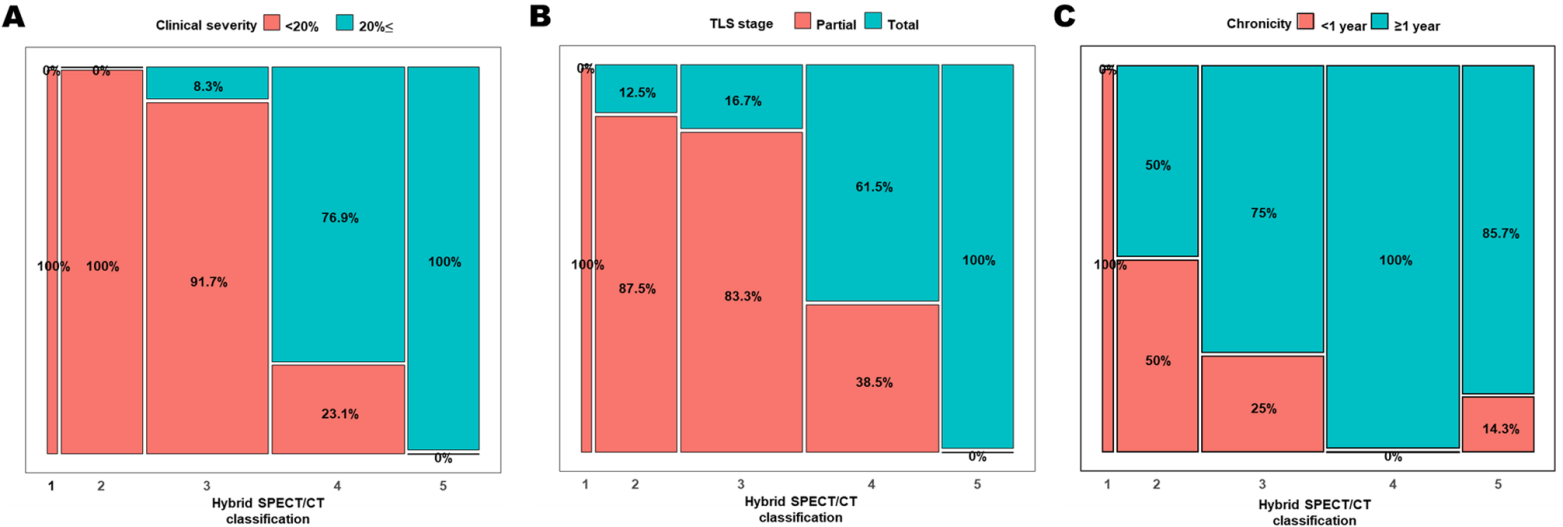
Changes of the hybrid SPECT/CT classification according to (A) clinical severity, (B) lymphoscintigraphic staging, and (C) chronicity.
